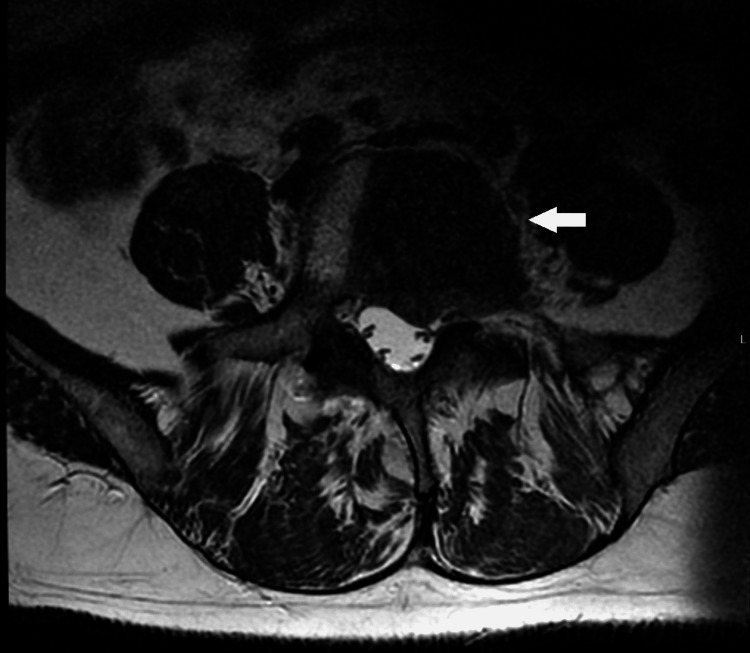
MRI, axial T2WI sequence. Axial T2WI highlights the existence of a left paravertebral soft tissue mass at L5, obliterating the left anterolateral aspect of the epidural space and the left L5-S1 foramen, probably with left L5 nerve root compression.
